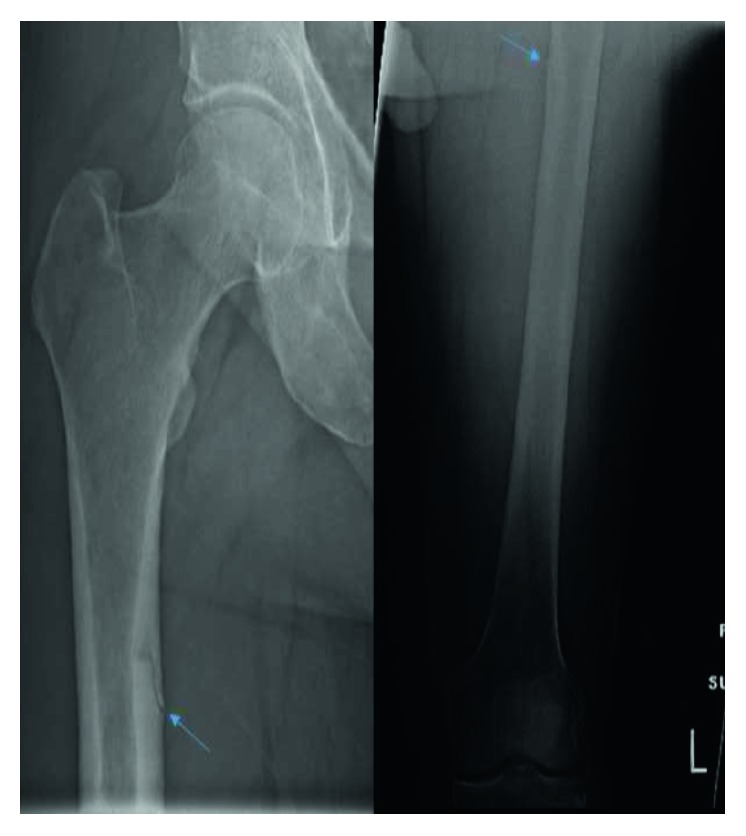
Radiographic image of both femurs with arrows pointing towards the fracture lines (the right femur on the left side and the left femur on the right side).
